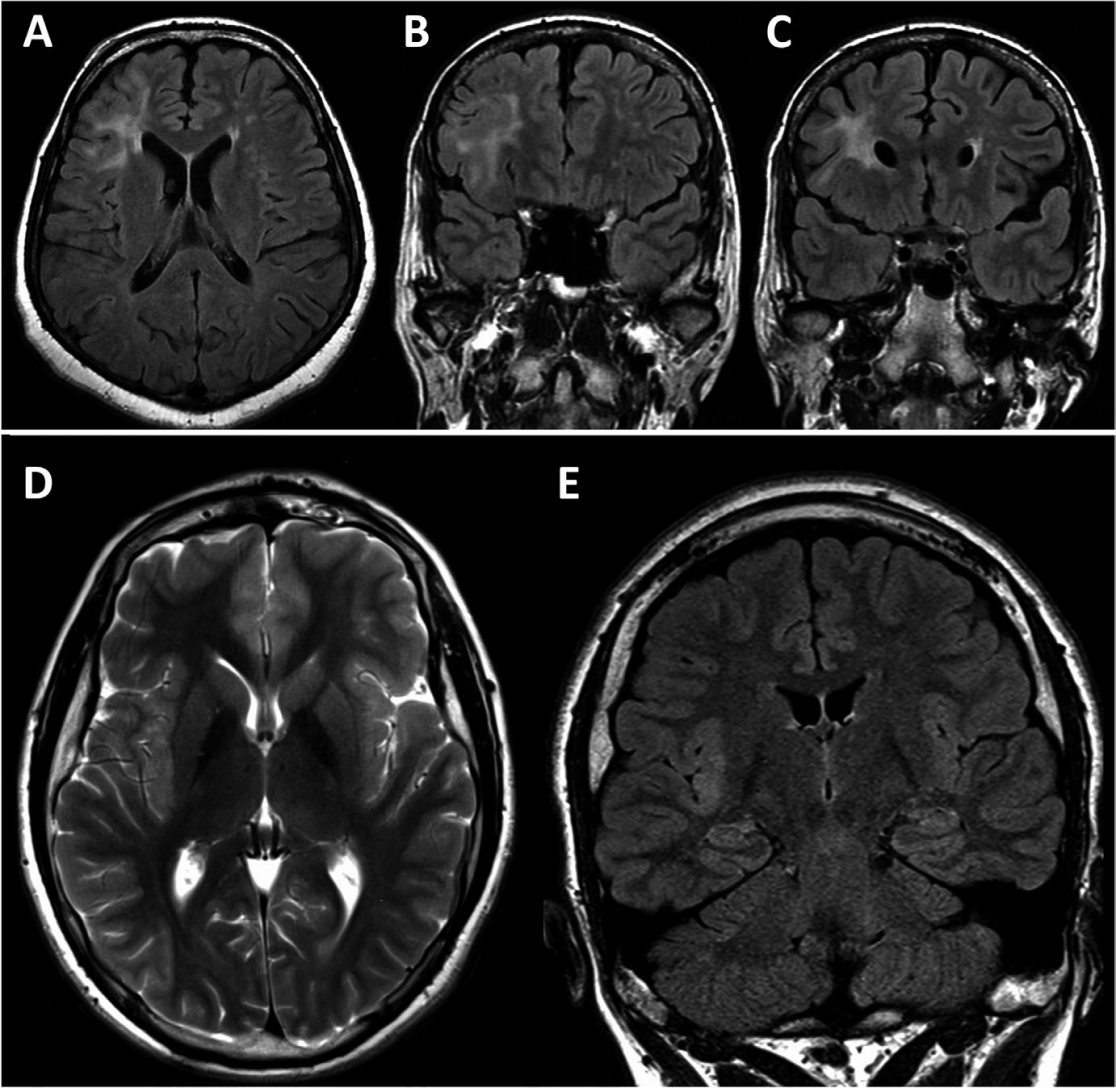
Presurgical MRI (A‐E) of two adult patients with focal cortical dysplasia type IIb. The first patient, a 38‐year‐old woman, had a large lesion involving the right frontal lobe as seen on axial and coronal planes of FLAIR (A‐C) images. In comparison, the lesion appears to be smaller and more localized to insular region on presurgical MRI including axial plane of T2‐weighted and coronal plane of FLAIR (D, E) images in the second patient, who is an 18‐year‐old boy. However, in this patient, invasive EEG revealed a wide epileptogenic zone involving the perirolandic area on dorsolateral and mesial surfaces
